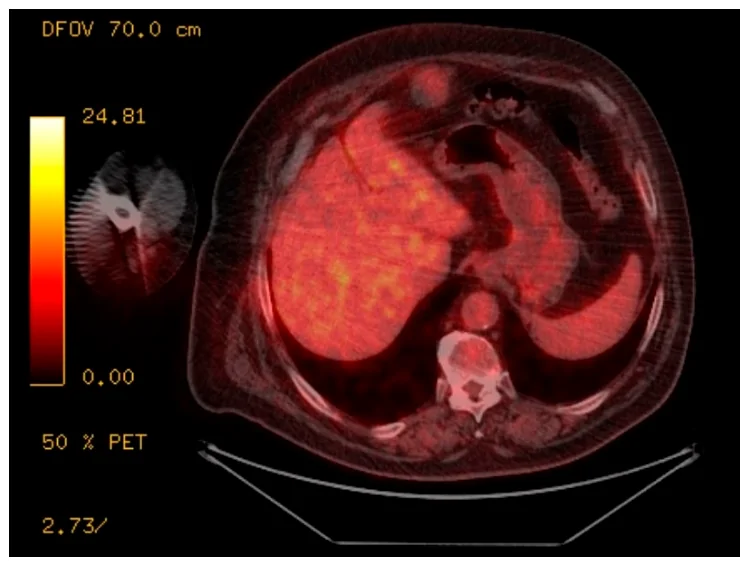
Preoperative axial fused PET/CT caption through the upper abdomen, inferior to the principal cardiac structures.
